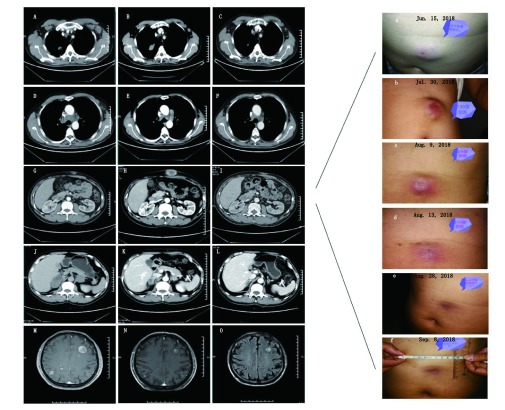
CT变化：基线水平（使用atezolizumab 2周前）；假性进展（治疗6周后）；肿瘤缩小（治疗12周后）。A-C：2018年8月3日胸部CT显示右肺上叶肿块（白色箭头）较基线水平明显增大。2018年9月13日，该肿块较前迅速缩小。治疗开始后左腋窝淋巴结持续缩小（红色箭头）；D-F：主动脉弓旁纵隔淋巴结（白色箭头）在治疗第6周时增大，第12周时明显减少。其余纵隔淋巴结体积在治疗后第6周显著减小（红色箭头）；G-I：前腹壁肿块（白色箭头）治疗第6周时出现，随后迅速增大，第12周时明显缩小；a-f：病人自行拍摄，前腹壁肿块肉眼外观变化，2018年7月30日该肿块最大；J-L：右肾上腺肿块第6周时明显缩小；第12周较第6周进一步缩小；M-O：第6周时脑转移肿块的数量及体积均减少，第12周较第6周变化不大 CT scans showing tumour response at baseline (2 weeks before initiation of atezolizumab), pseudoprogression (immediately after 6 weeks of treatment), and after tumour shrinkage (after 12 weeks of treatment). A-C: Chest CT images show the right superior lobe mass (white arrow) significantly increased in size on August 3, 2018 compared with the baseline. On September 13, 2018, the lesion shrunk significantly. Left axillary lymph nodes decreased in size since therapy (red arrow). D-F: The mediastinal lymph nodes (white arrow) near the aortic arch grew larger at week 6 and subsequently decreased definitely at week 12. Others significantly decreased their size at week 6 (red arrow). G-I: An anterior abdominal wall mass (white arrow) was detected at week 6, which was larger and subsequently smaller at week 12. a-f: The size change of the anterior abdominal wall mass, photographed by the patient himself. The anterior abdominal wall masssignificantly increased in size on July 30, 2018. J-L: Right adrenal mass shrunk significantly at week 6. M-O: The amount and volume of brain metastasis were both reduced at week 6 and week 8
